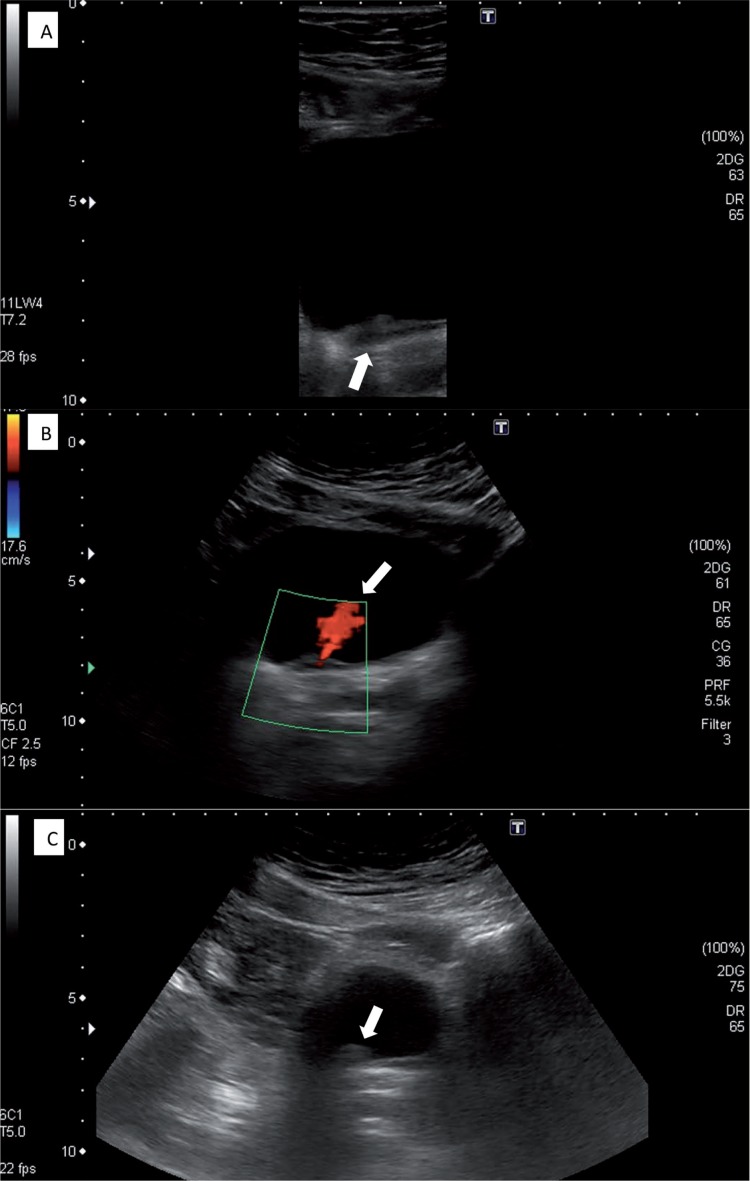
A: US with an area of focal thickening in the bladder floor (arrow); B: The focal thickening in the bladder floor is close to the ureteral meatus (arrow show the ureteric jet in US doppler); C: Nodular lesion in the bladder floor, close to the focal thickening (arrow).
